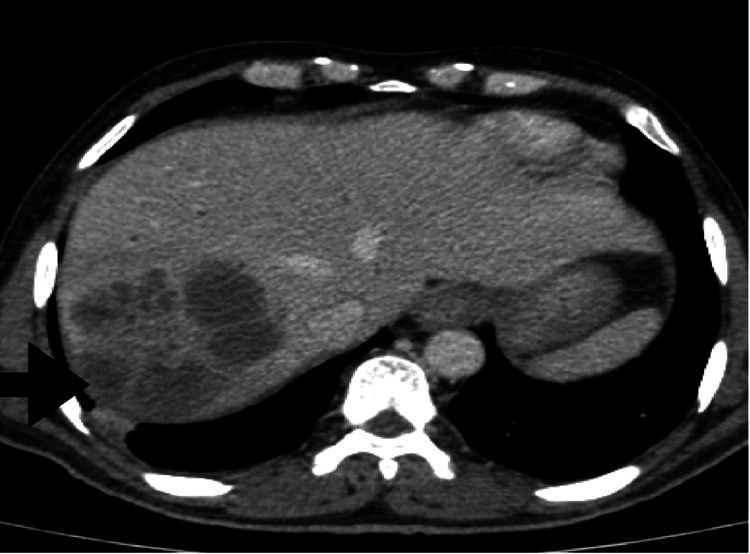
Contrast-enhanced computed tomography of the abdomen showing multiloculated hypodense lesion with multiple internal septations measuring 6.9 x 7.4 x 7.5 cm (anteroposterior x transverse x craniocaudal, respectively) with a total volume of 383 cc.
